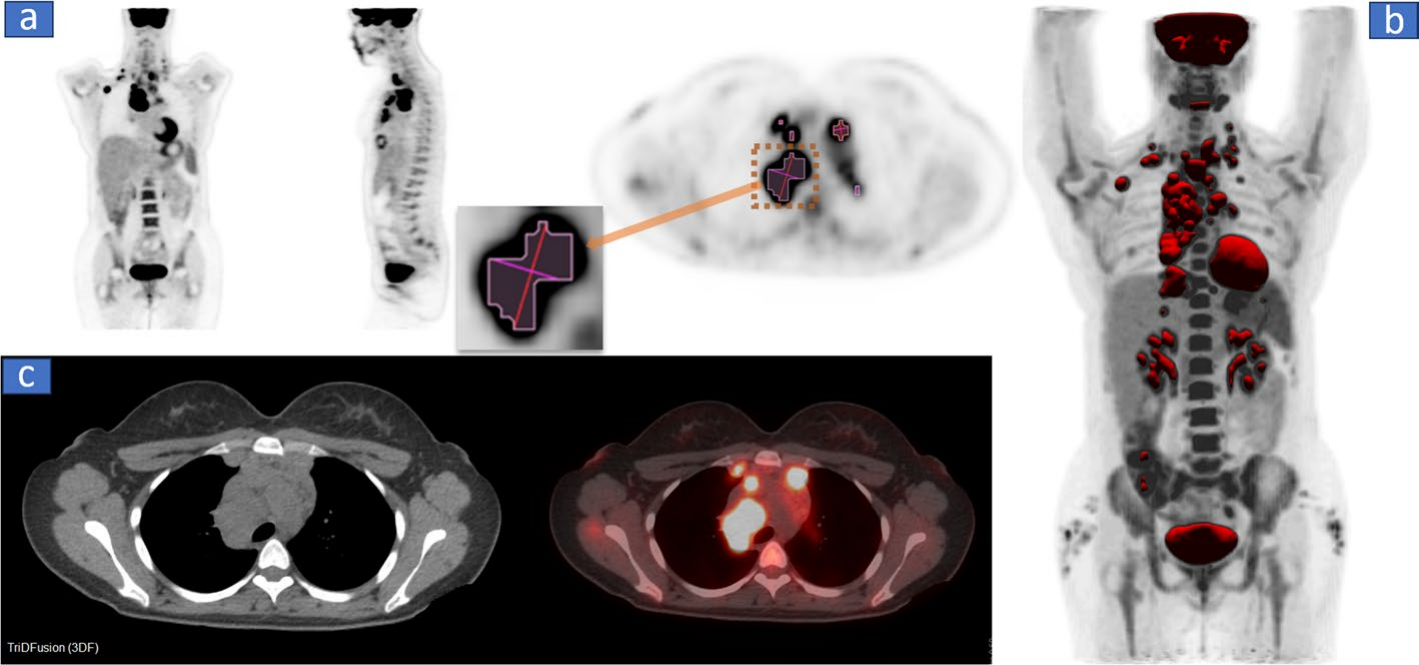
a The standard coronal, sagittal, and axial positron emission tomography (PET) displays in grayscale with segmented volumes of interest. The maximum intensity projection displayed in b is fused with the isosurfaces. Note the RESIST distance tool shown in the exploded view. c The transaxial computed tomography (CT) and PET/CT fusion images
